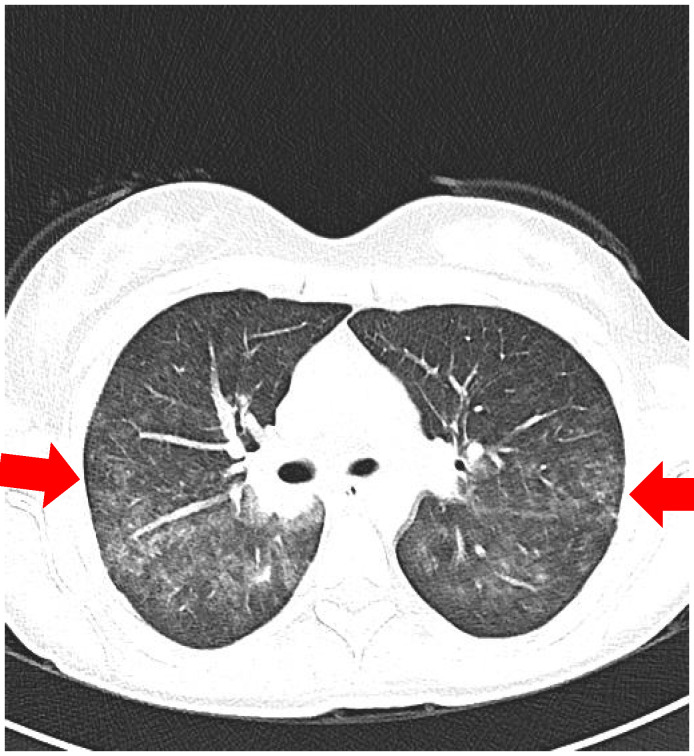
Thoracic HRCT (lung window): Axial image demonstrating bilateral ground-glass opacities with smooth interlobular septal thickening (“crazy-paving”) and multiple peribronchovascular nodules (right > left).
